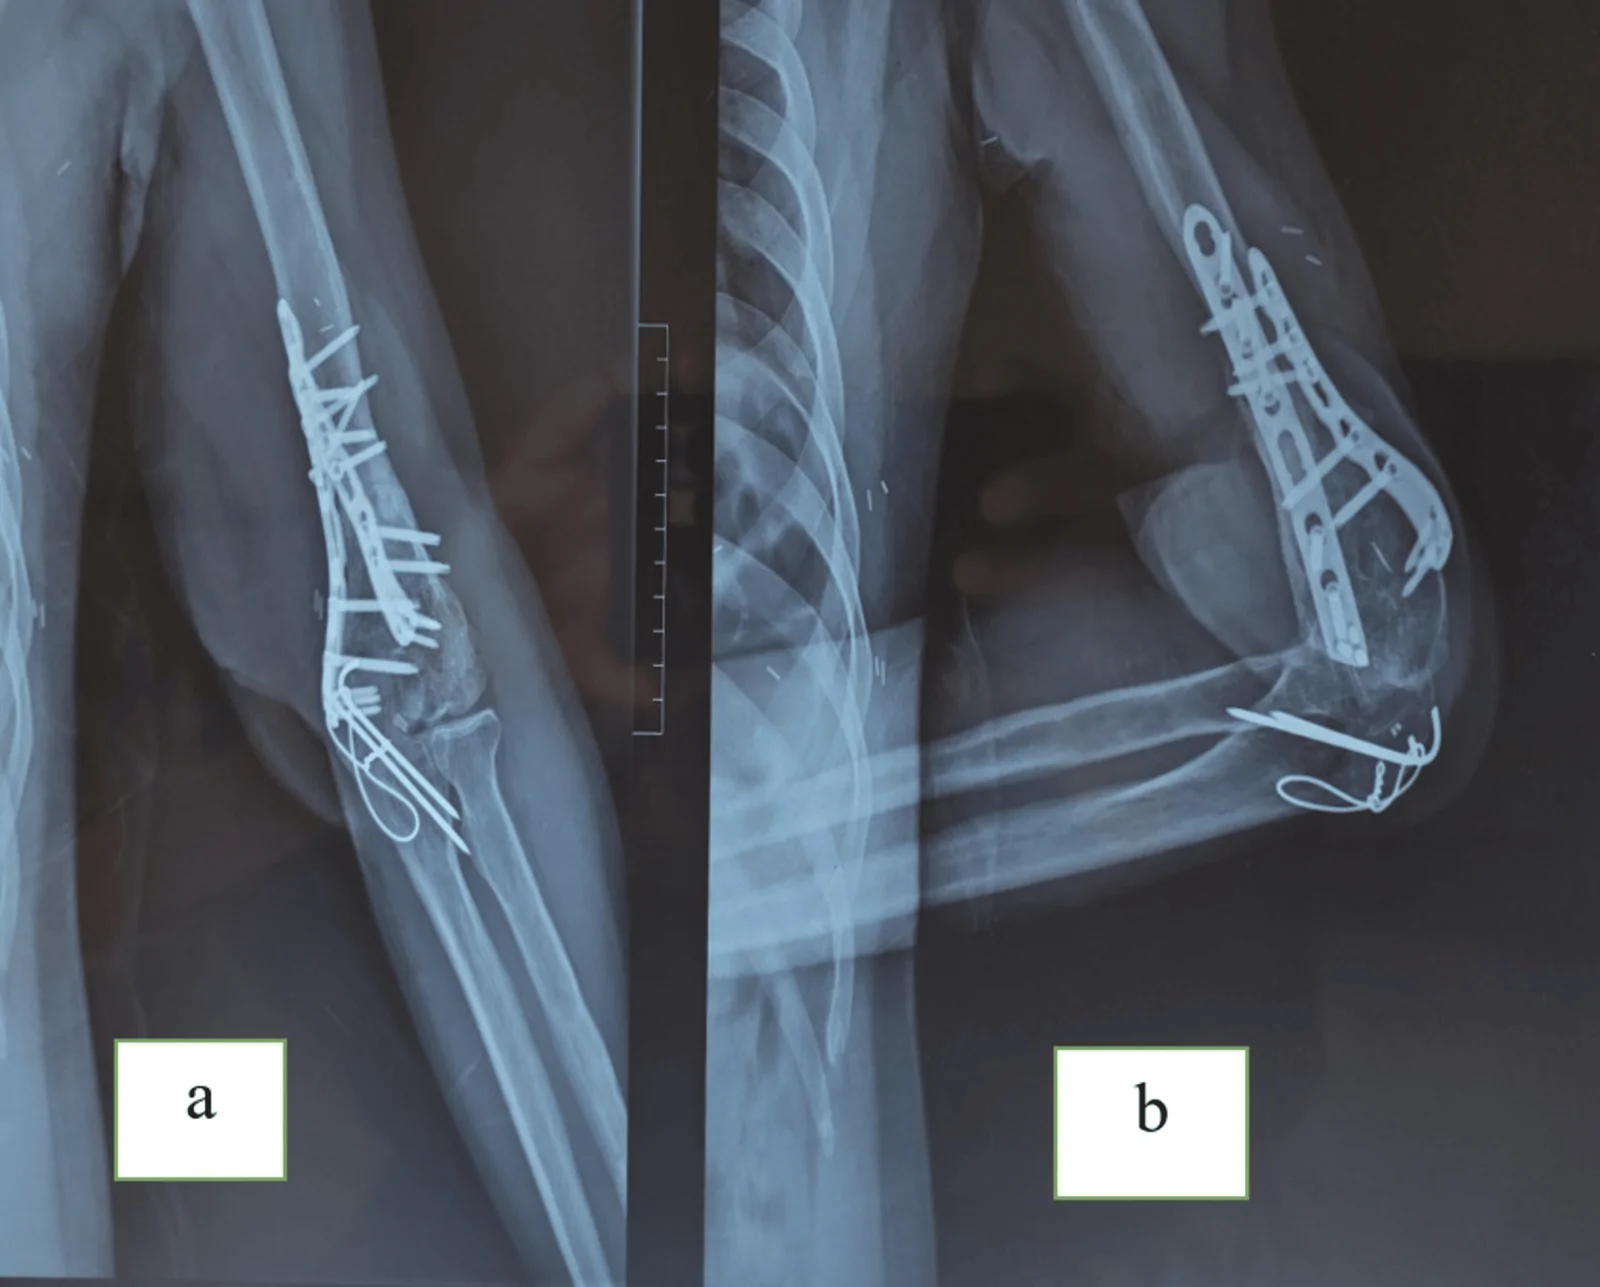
Follow-up X-ray after one year
